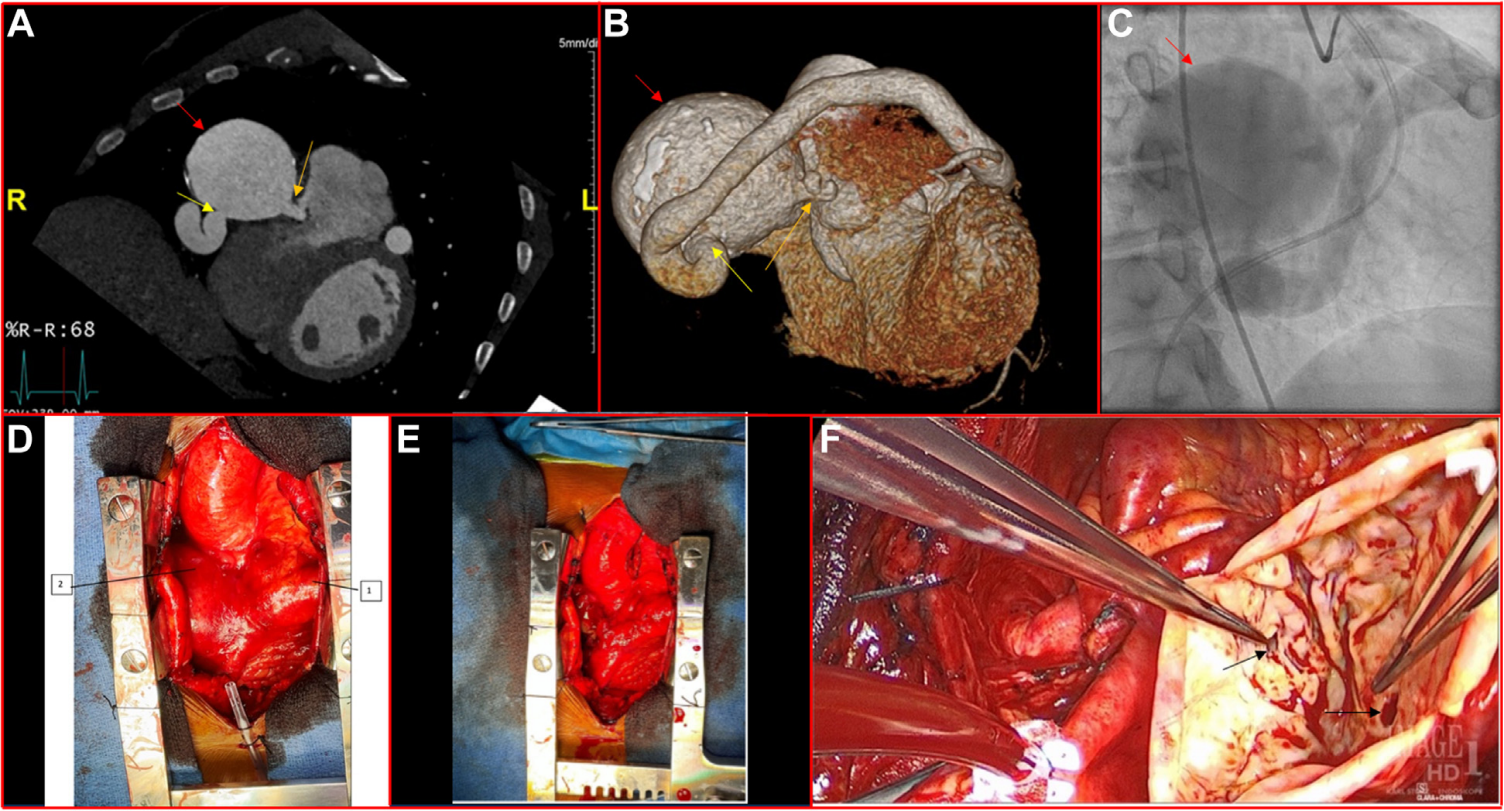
Coronary CTA, Cardiac Catheterization, and Intra-operative Images (A) Computed tomography angiography of the heart and coronary arteries demonstrating the contained rupture (red arrow) with connection to the right ventricle (orange arrow) and connection to the aneurysmal single coronary artery (yellow arrow). (B) Three-dimensional reconstruction of coronary computed tomography angiography redemonstrating contained rupture and connections to the right ventricle and aneurysmal single coronary artery. (C) Left heart catheterization image demonstrating aneurysmal single coronary artery and contained rupture. (D) Intraoperative image demonstrating aneurysmal single coronary artery (1) feeding into contained rupture (2). (E) Intraoperative image after arteriotomy and ligation. (F) Opened contained rupture with connections to right ventricle and coronary artery indicated by forceps and solid arrows.
